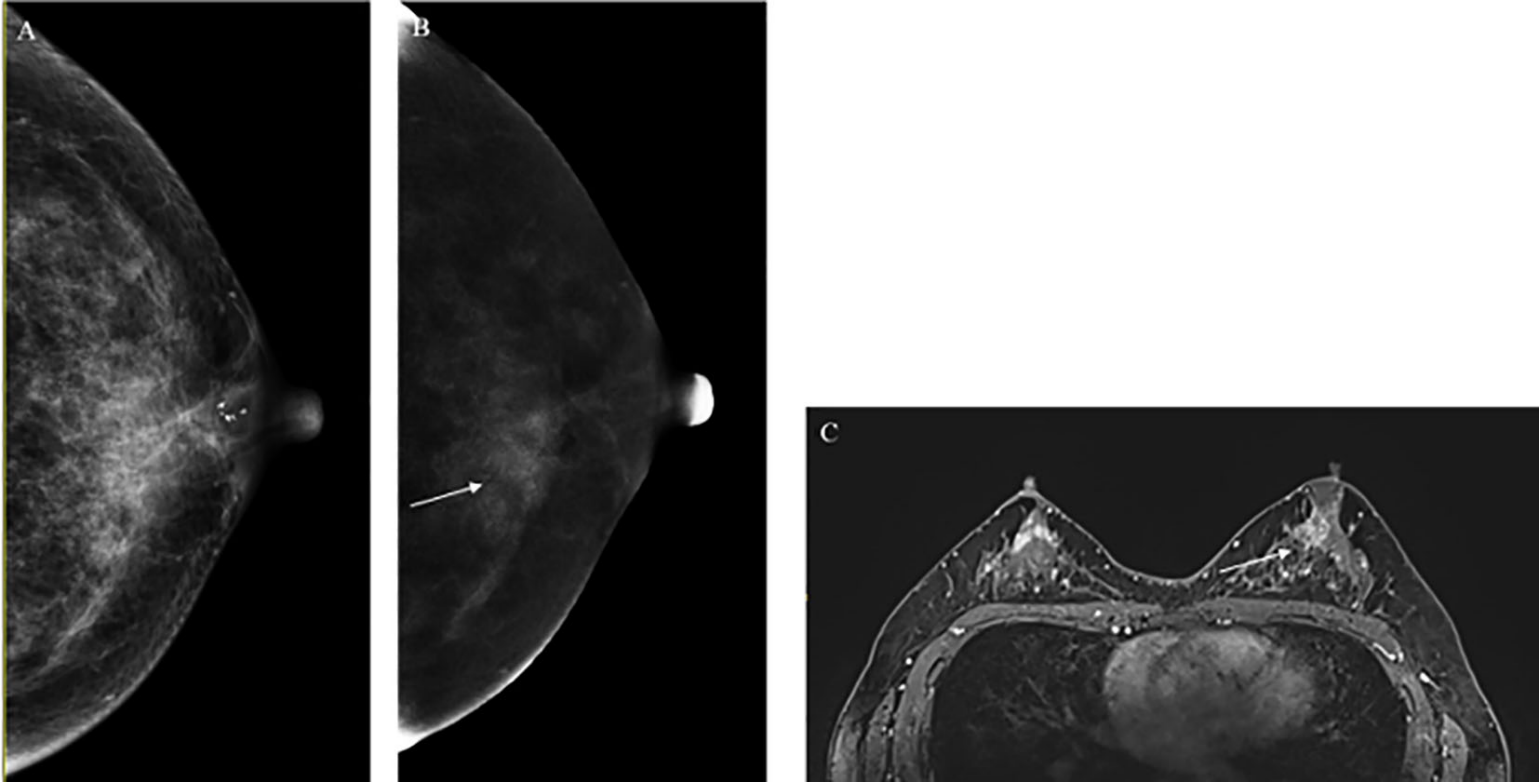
Radiological cases in which both CEM and MRI incorrectly identified benign lesions as malignant. (A) CEM CC low-energy image; (B) CEM CC subtraction image: A small, patchy isodense lesion (indicated by the white arrow) is observed in the inner upper quadrant of the left breast, with irregular edges and enhancement upon contrast administration; (C) MRI T1-weighted enhanced image: A planar, irregularly enhanced focus is seen in the left nipple region. Both imaging modalities suggest a tumor-like lesion, classified as BI-RADS 4C. Pathological findings revealed a papillary tumor with ductal epithelial hyperplasia.
